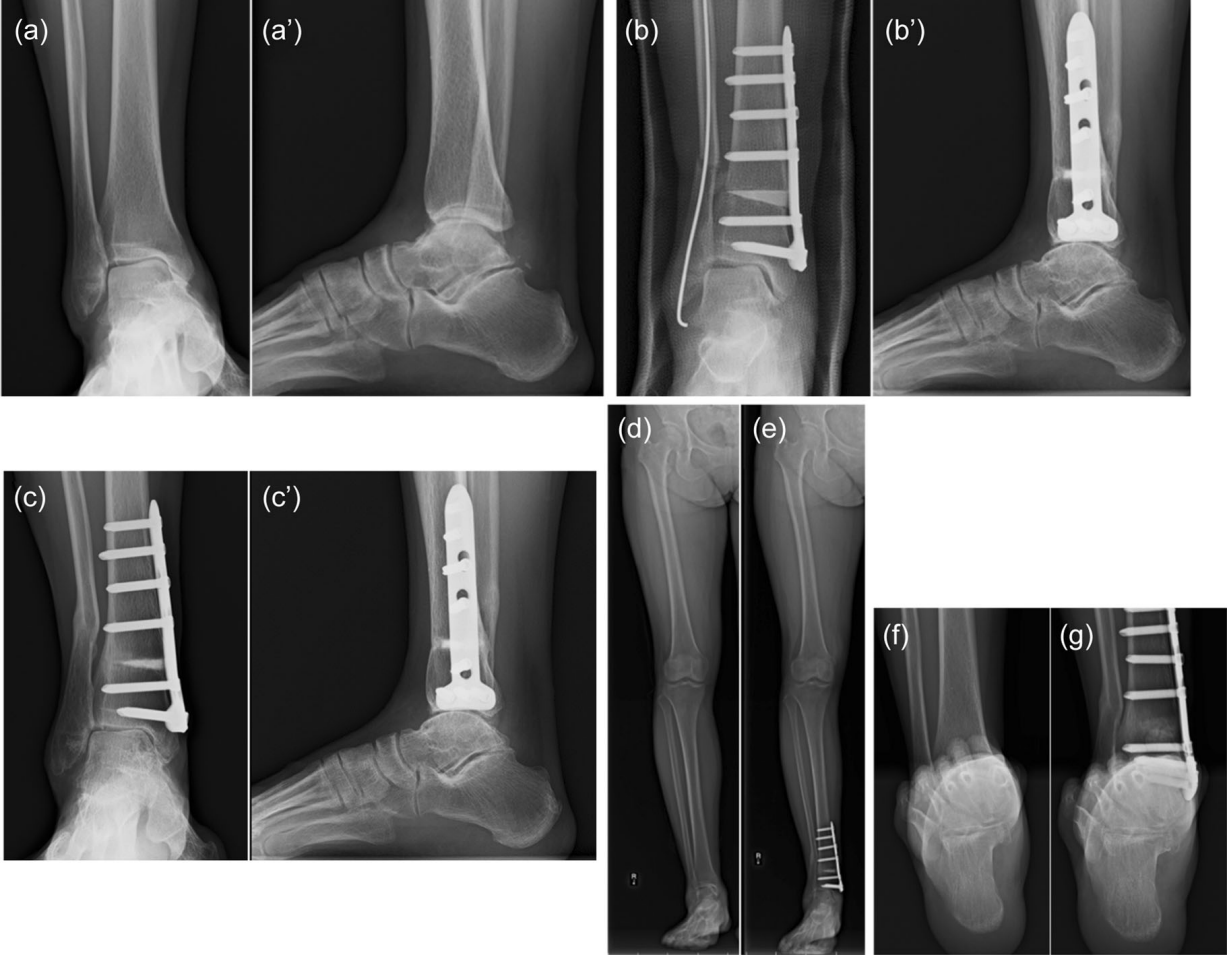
Case: 58‐year‐old female. (a and a') Preoperative radiograph of the frontal and lateral ankle joints. (b and b') Postoperative radiograph of the frontal and lateral ankle joints acquired immediately after the surgery. (c and c') Radiograph of the frontal and lateral ankle joints acquired 1 year postoperatively. (d) Radiograph of the whole leg standing radiography of preoperatively. (e) Radiograph of the whole leg standing radiography of 1 year postoperatively. (f) Weightbearing Subtalar radiograph acquired preoperatively. (g) Weightbearing Subtalar radiograph acquired 1 year postoperatively.
